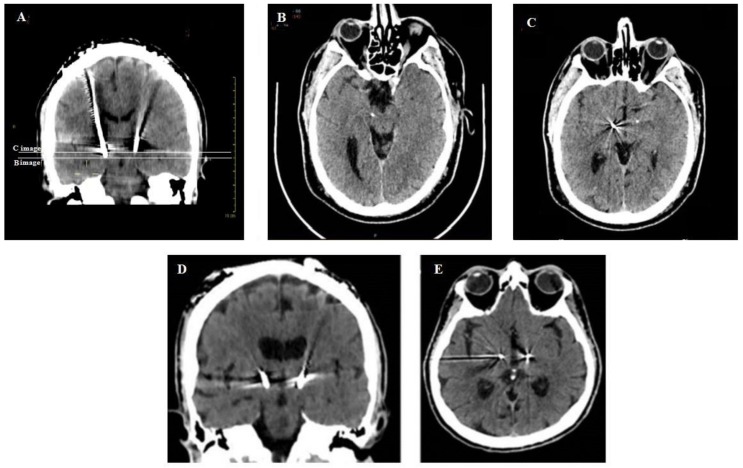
A representative comparison in postoperative CT scans of a patient implanted without intraoperative X-rays control (A–C) with a patient implanted with intraoperative X-rays control (D,E). The coronal CT-scan shows the different depth of electrodes in the STN; the white lines correspond to the axial levels of the CT scan reported in B and C. The distal tips of the electrode in the right and left STN are visible in B and C, respectively. The contact 1 of the electrode in the right STN and the distal tip of the electrode in the left STN are visible in C. The correct trajectory of the two electrode and the precise correspondence of their tips in a patient implanted under X-ray control are visible in the coronal (D) and axial (E) CT scans.
